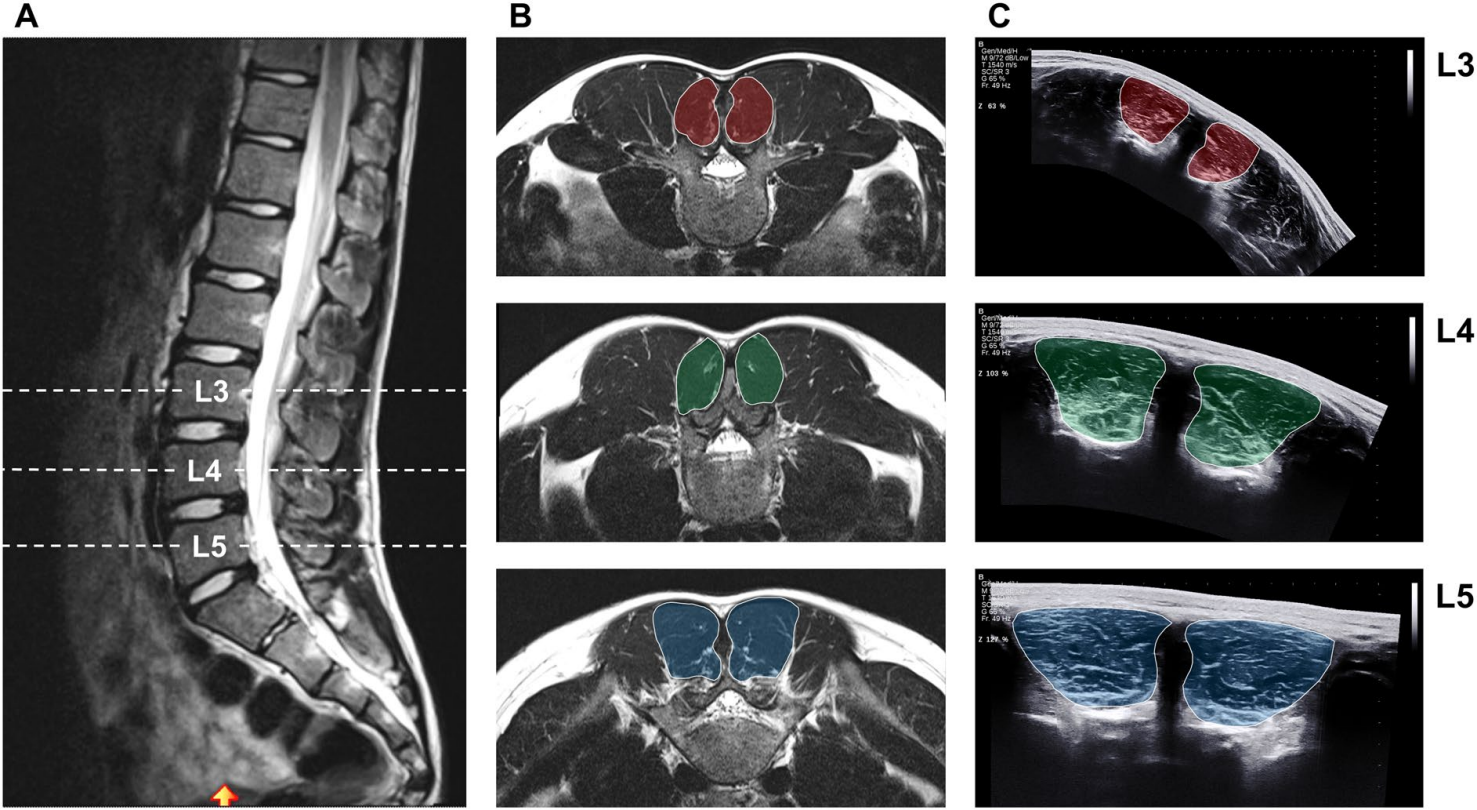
Representative MRI and US images of the LMF ACSA at lumbar vertebral bodies L3-L5. (A) Sagittal MRI image with identified lumbar vertebral bodies L3-L5; (B) Transversal MRI images of LMF ACSA at L3, L4 and L5; (C) Transversal panoramic US images of LMF ACSA at L3, L4 and L5. *MRI* magnetic resonance imaging, *US* ultrasound, *LMF ACSA* lumbar multifidus anatomical cross-sectional area.
